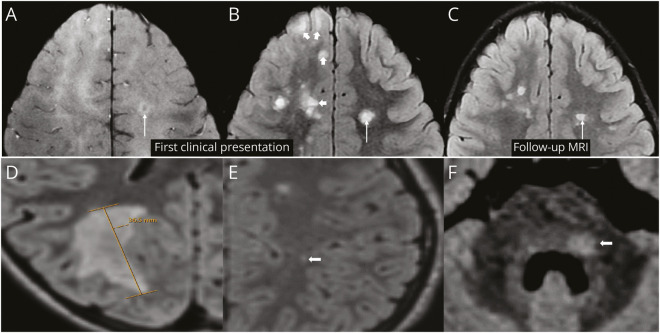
Persisting and Resolving Lesions Axial SWI (A) and FLAIR (B) images reveal multiple focal lesions in the white matter. Among these, a lesion in the left frontal deep white matter (long arrow, B) demonstrates the CVS on SWI (long arrow, A), while the remaining lesions (small arrows, B) lack the CVS. Follow-up imaging shows the resolution of most CVS-negative lesions, with the CVS-positive lesion (arrow, C) persisting. D, E, and F show examples of lesions excluded from analysis. Axial FLAIR images show a large confluent lesion >2 cm in size (D), a punctate lesion <3 mm in size (arrow, E), and a brainstem lesion (arrow, F). CVS = central vein sign; FLAIR = fluid-attenuated inversion recovery; SWI = susceptibility-weighted imaging.
